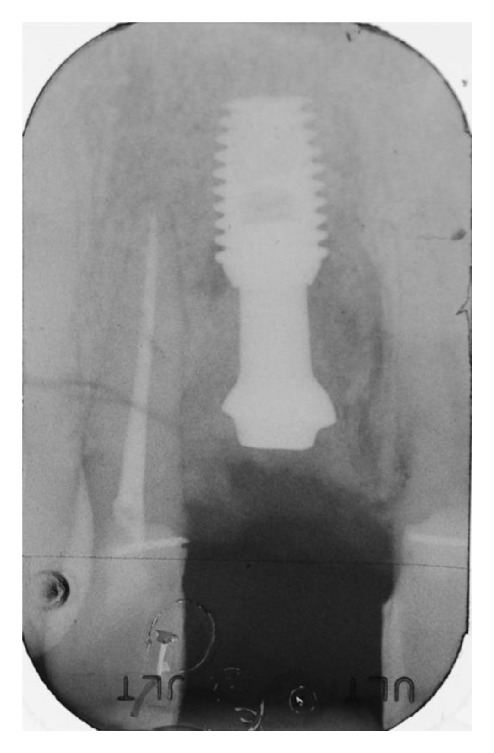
Postoperative X-ray shows fulfilling of the defect, with the implant lying in native bone below the grafted material.
